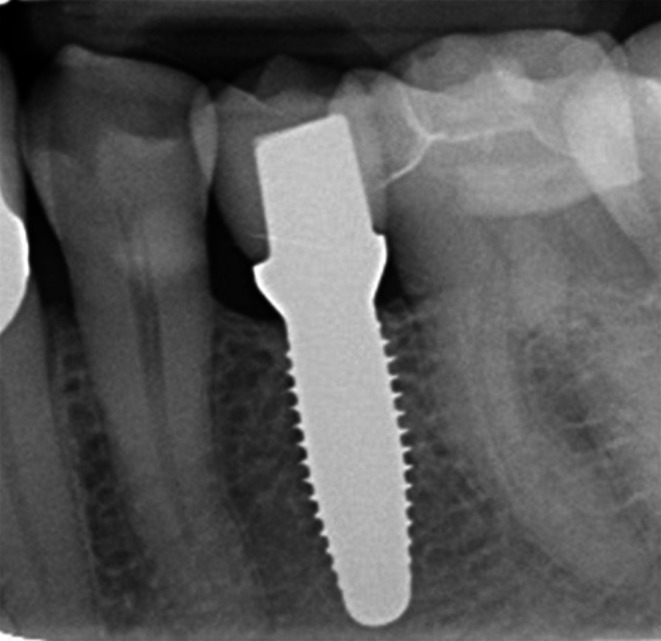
Corresponding radiograph of the peri‐implant bone situation of the SC implant at the 10‐year follow‐up.
